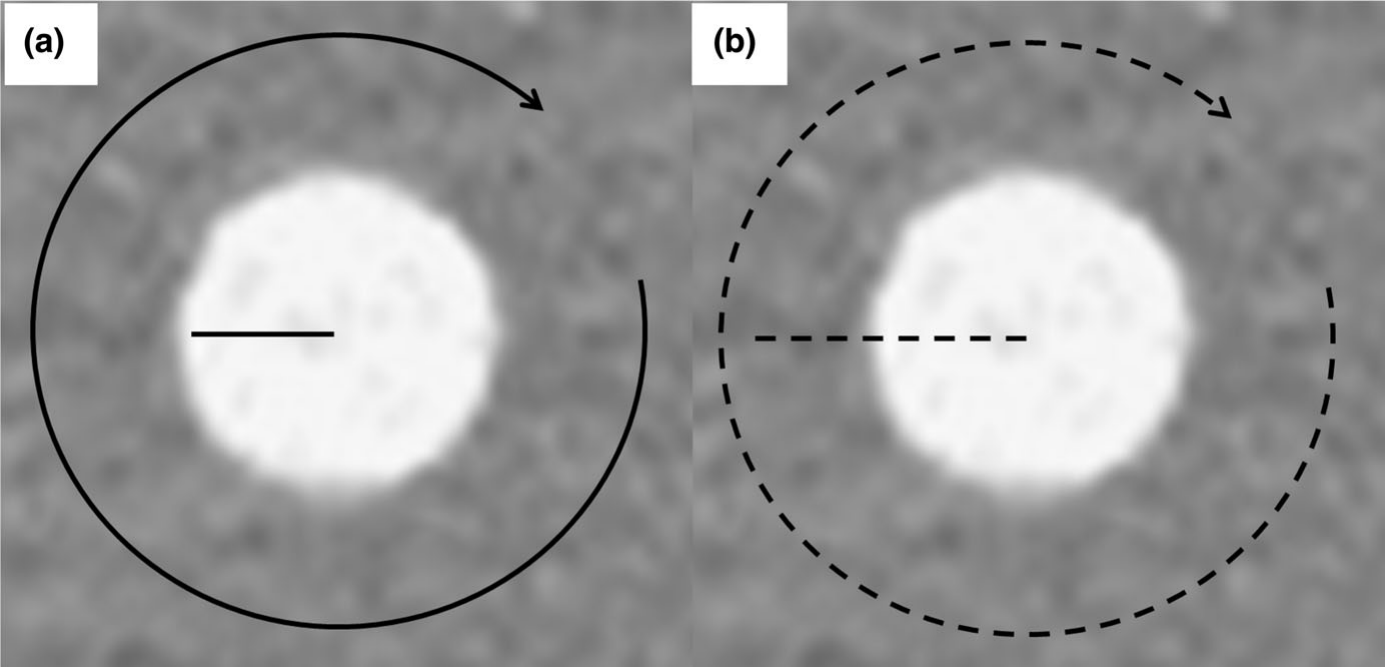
Alignment of the line ROI to record the CT number and attenuation profile curves. The CTP 404 module was used to record the CT number and attenuation profile curves of the high‐, moderate‐, and low‐signal objects. (a) The CT numbers of these objects for each reconstruction algorithm were recorded radially around the object centers at 1° intervals using a line region of interest (1 × 27 pixels, 5‐mm circle‐radius). (b) The attenuation profile curves of these objects for each reconstruction algorithm were recorded radially around the object centers at 1° intervals using a line region of interest (1 × 51 pixels, radius of the circle of 10 mm).
